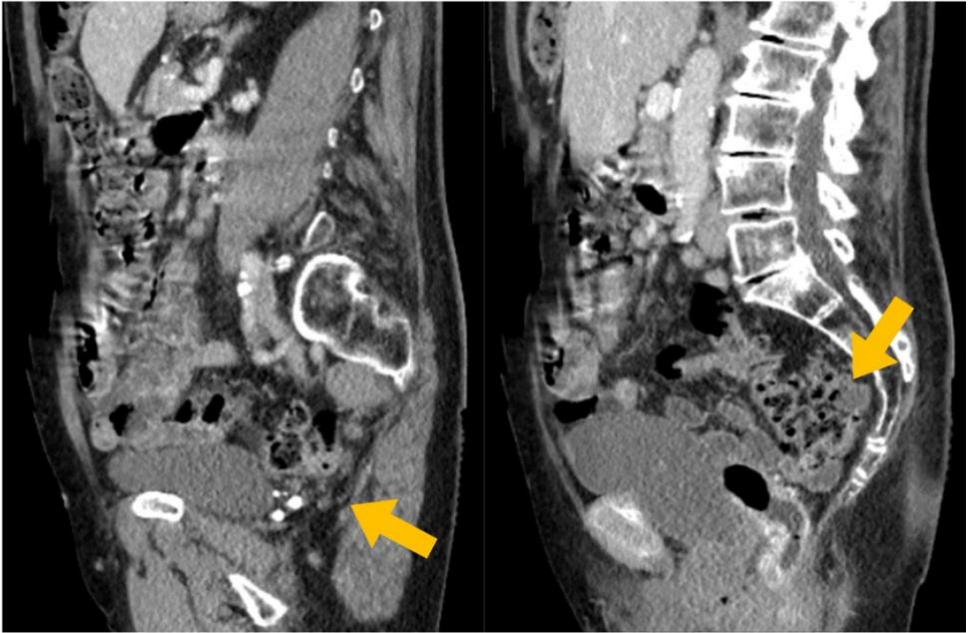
Radiologic imaging prior to surgery. The abdominal CT scan showed the relevant and, in most part, retrorectal elongation of the sigmoid colon in the two sagittal pictures (yellow arrows).
